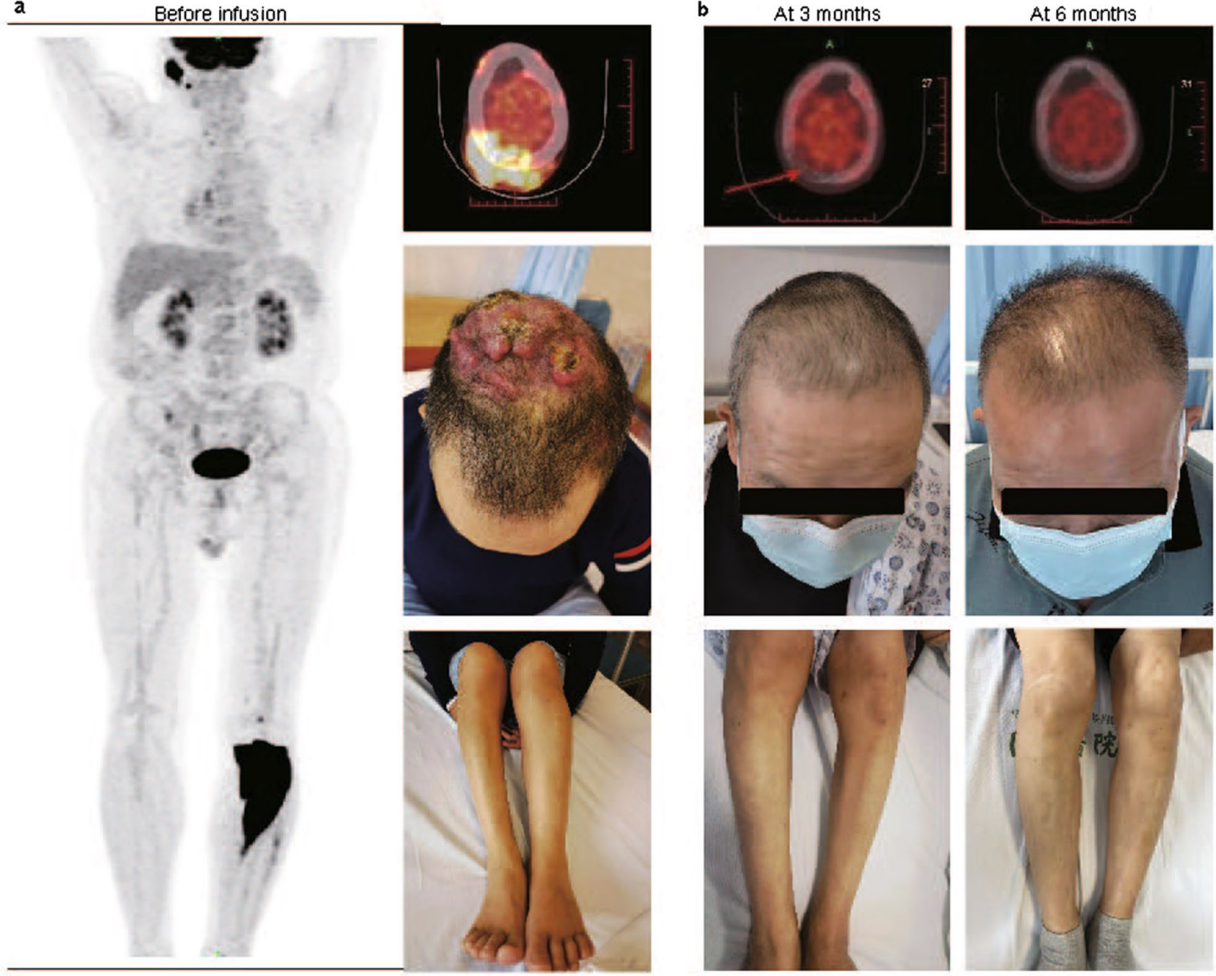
Extramedullary lesions in patient 1 before and after chimeric antigen receptor (CAR) -T cells infusion. a The results of combined positron emission tomography and computed tomography (PET-CT) with 18F-fluorodeoxyglucose demonstrated increased glucose metabolic activity in the calvaria and tibia of patient 1 before CAR-T infusion (left and up right). The gross appearance of soft tissue masses in the calvaria (right middle) and tibia (bottom right) were shown. b No lesion was observed for patient 1 by physical examination and PET-CT at 3 months and 6 months after infusion
